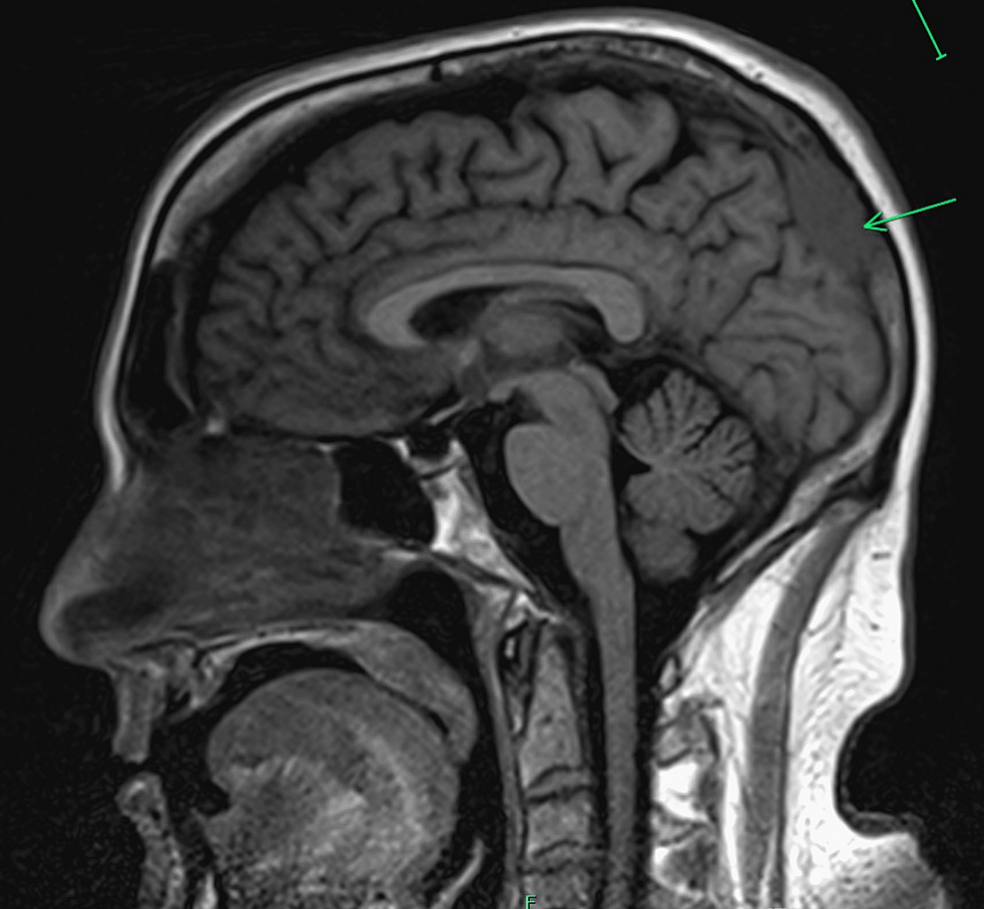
Magnetic Resonance Imaging (MRI) of the brain demonstrating bony metastatic deposit to the superior occipital bone measuring up to 3.2 x 1.6 x 2.1 cm with involvement of the dura. No vasogenic edema within the underlying brain parenchyma in the region of the bony metastatic deposit.
